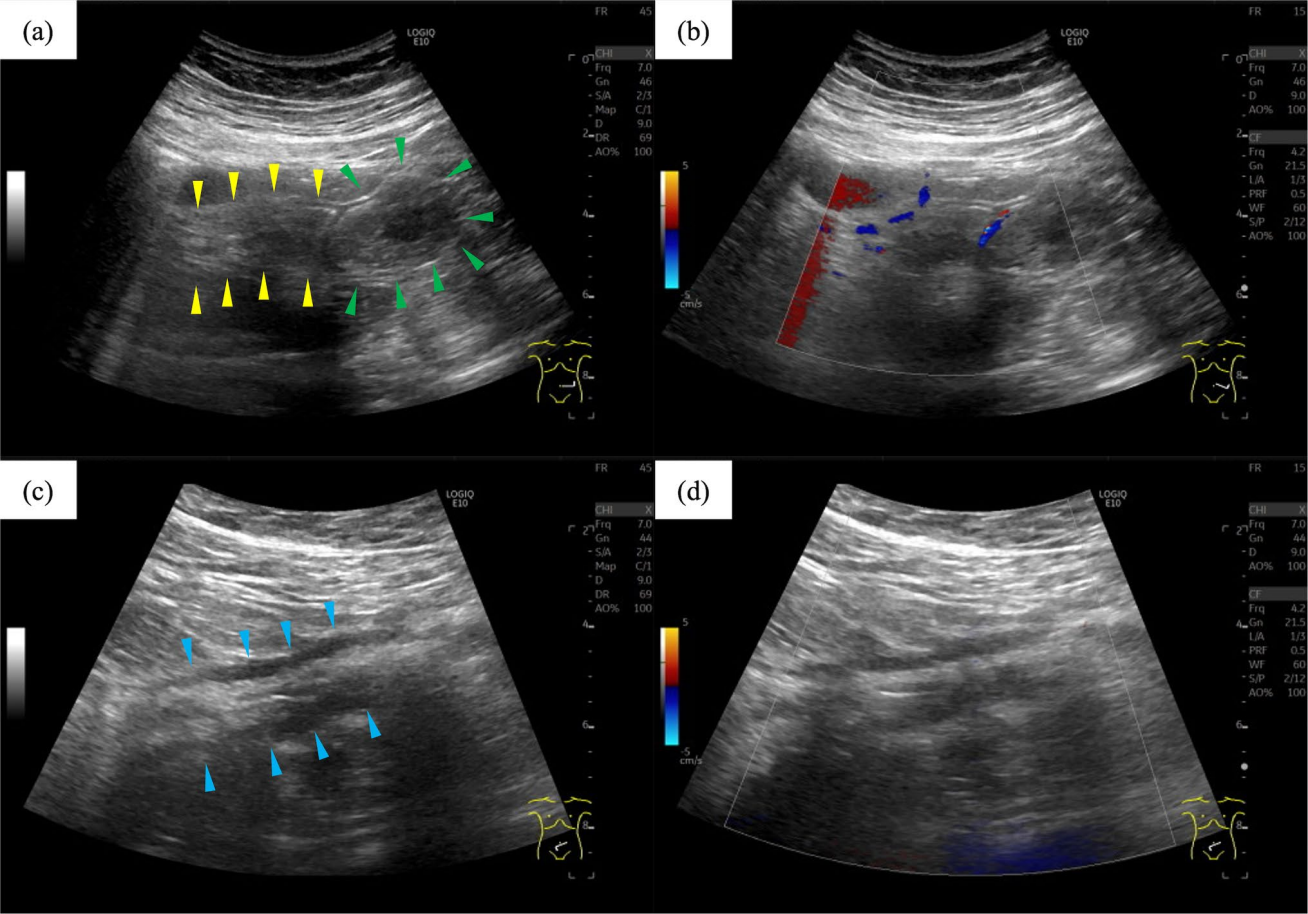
Intestinal ultrasonography image at the time of exacerbation. a The jejunum in the long axis image and the short axis image were indicated by the yellow and green arrowheads, respectively. Intestinal wall thickening was noted. b Color Doppler showed that blood flow was increasing on the serosal side of the jejunum (velocity range: 5 cm/second). c The sigmoid colon in the long axis image is indicated by the blue arrow. Intestinal wall thickening was observed. d Color Doppler showed no blood flow signals in the sigmoid colon (velocity range: 5 cm/second)
